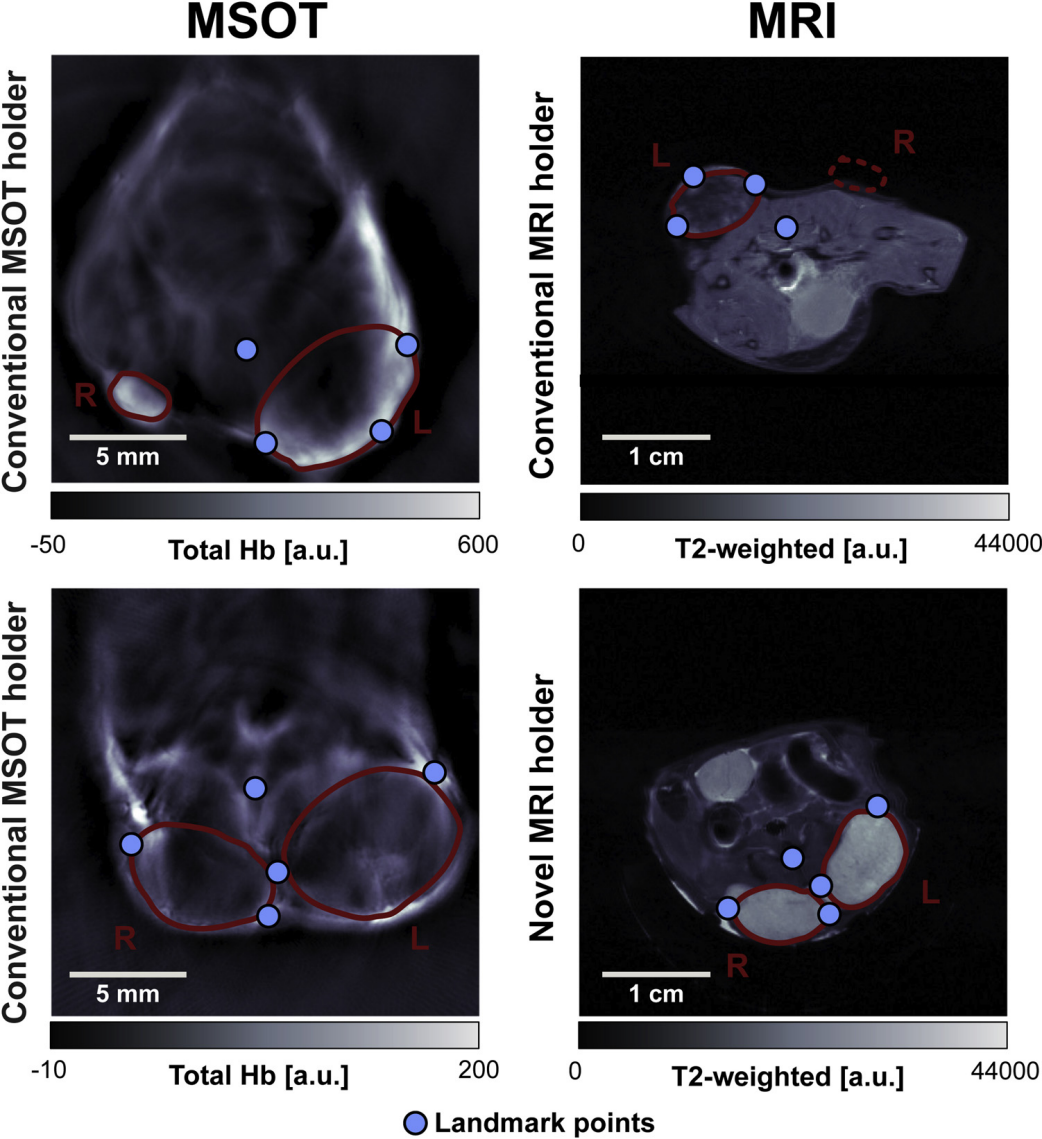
Qualitative comparison of tomographic image data from OT and MRI modalities using the conventional and novel MRI holders. *Top*: MSOT/MRI image pair with conventional holder geometry as in Fig. 1. Tumour R is not visible in the plane of the corresponding MR image. *Bottom*: MSOT/MRI image pair with novel holder geometry as in Fig. 2.
